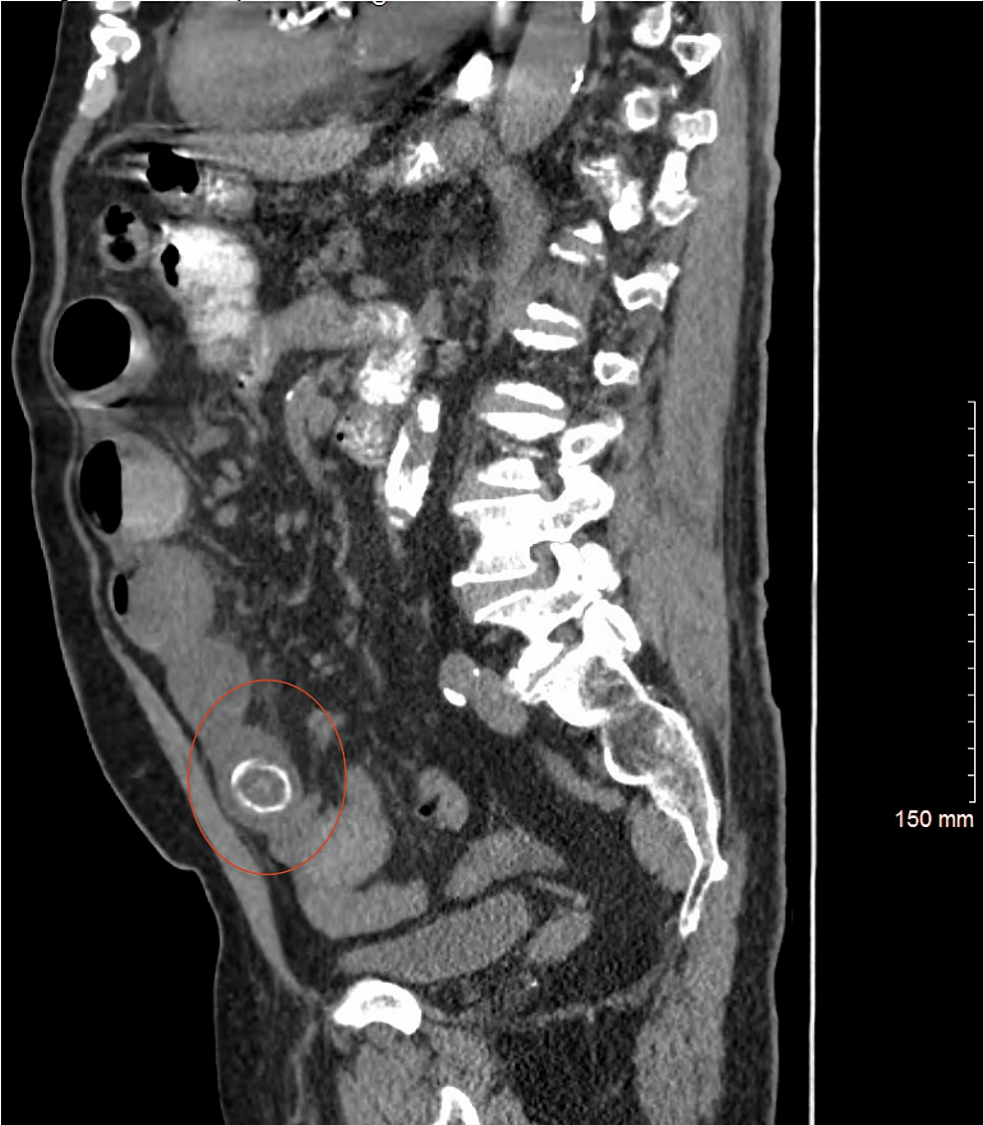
Sagittal CT abdomen and pelvis showing the dilated small bowel loops and impacted gallstone in the jejunum (red circle).
